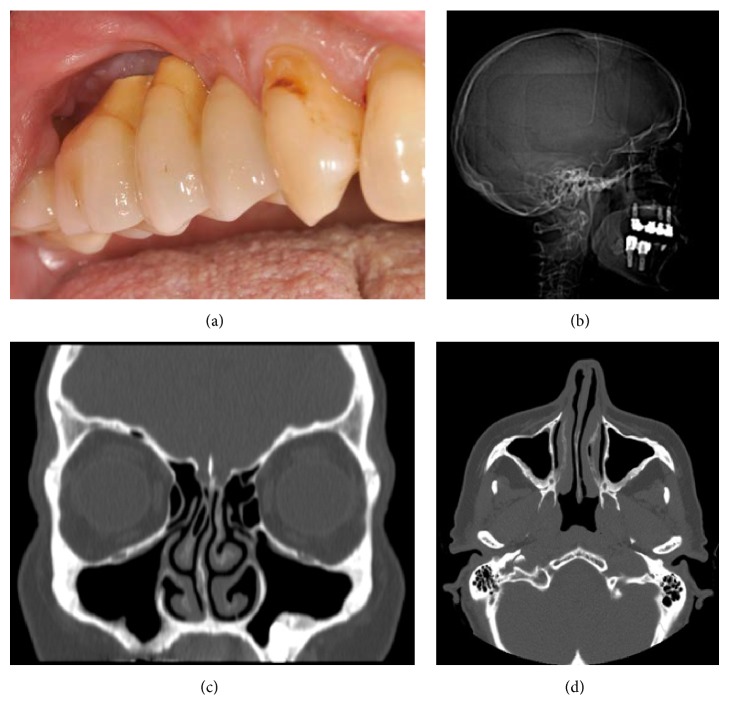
(a) Clinical view of the healed surgical area. (b) Lateral cephalogram without any sign of the foreign body into the sinus. (c, d) The CT scans show normal mucosal thickness and no opacification of the right maxillary sinus.
